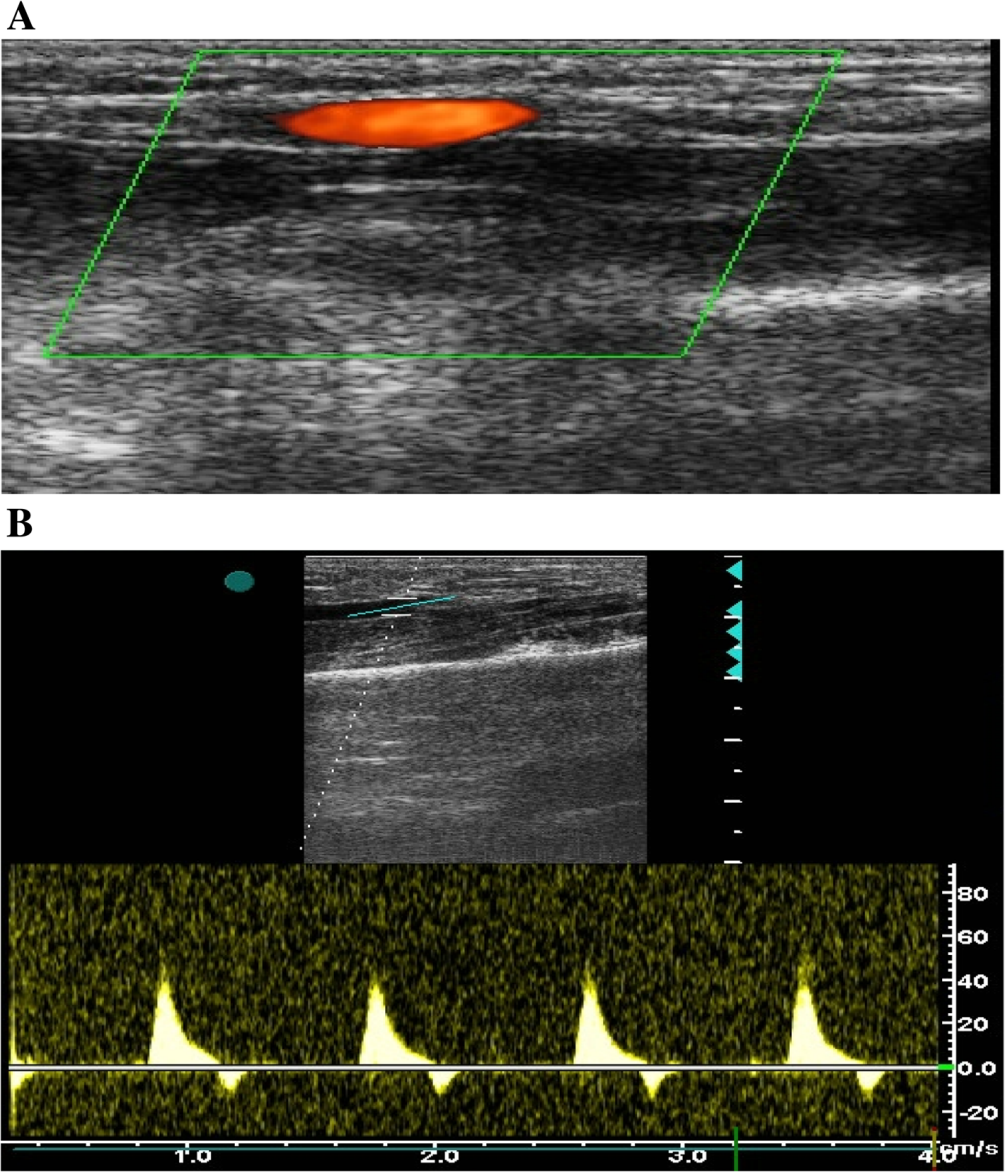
Duplex ultrasonography of a patent RA after TR coronary catheterization. a Color Doppler showing normal flow within the RA. b Spectral analysis showing tri-phasic flow pattern
